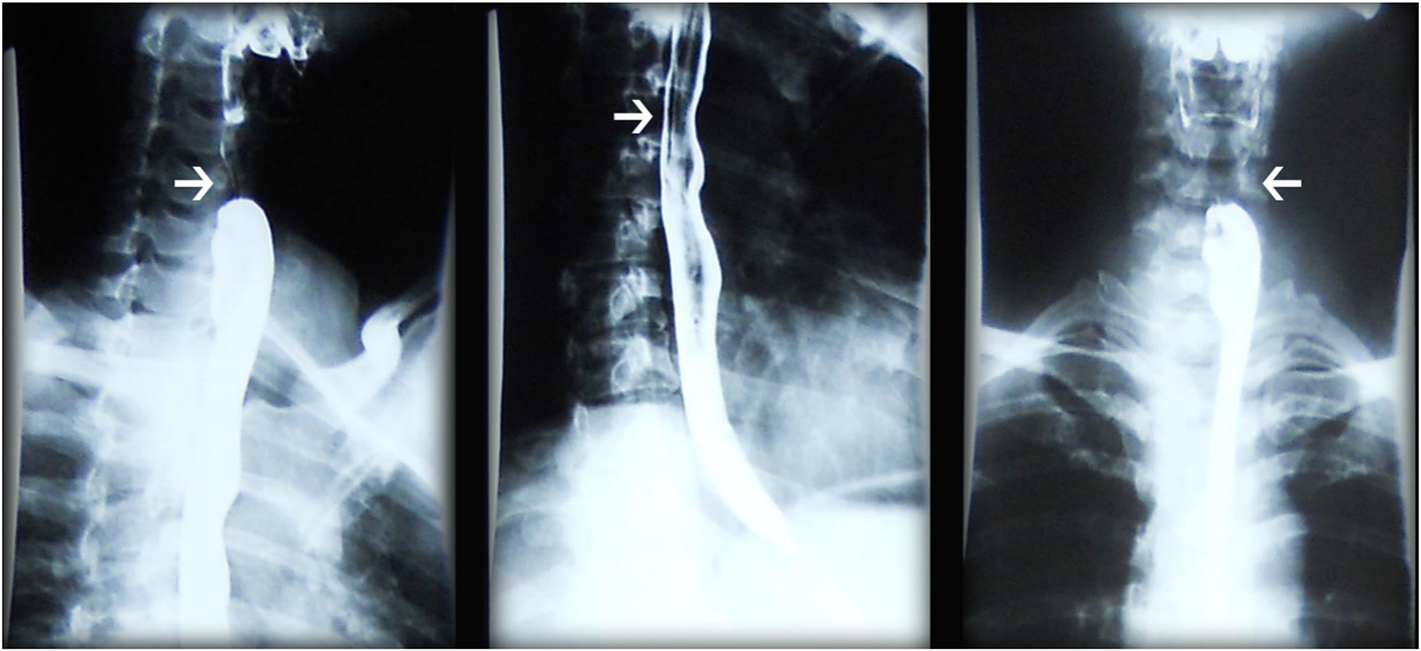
X-ray showed compression of the esophagus. The white arrow indicates the compressed part of the esophagus.
